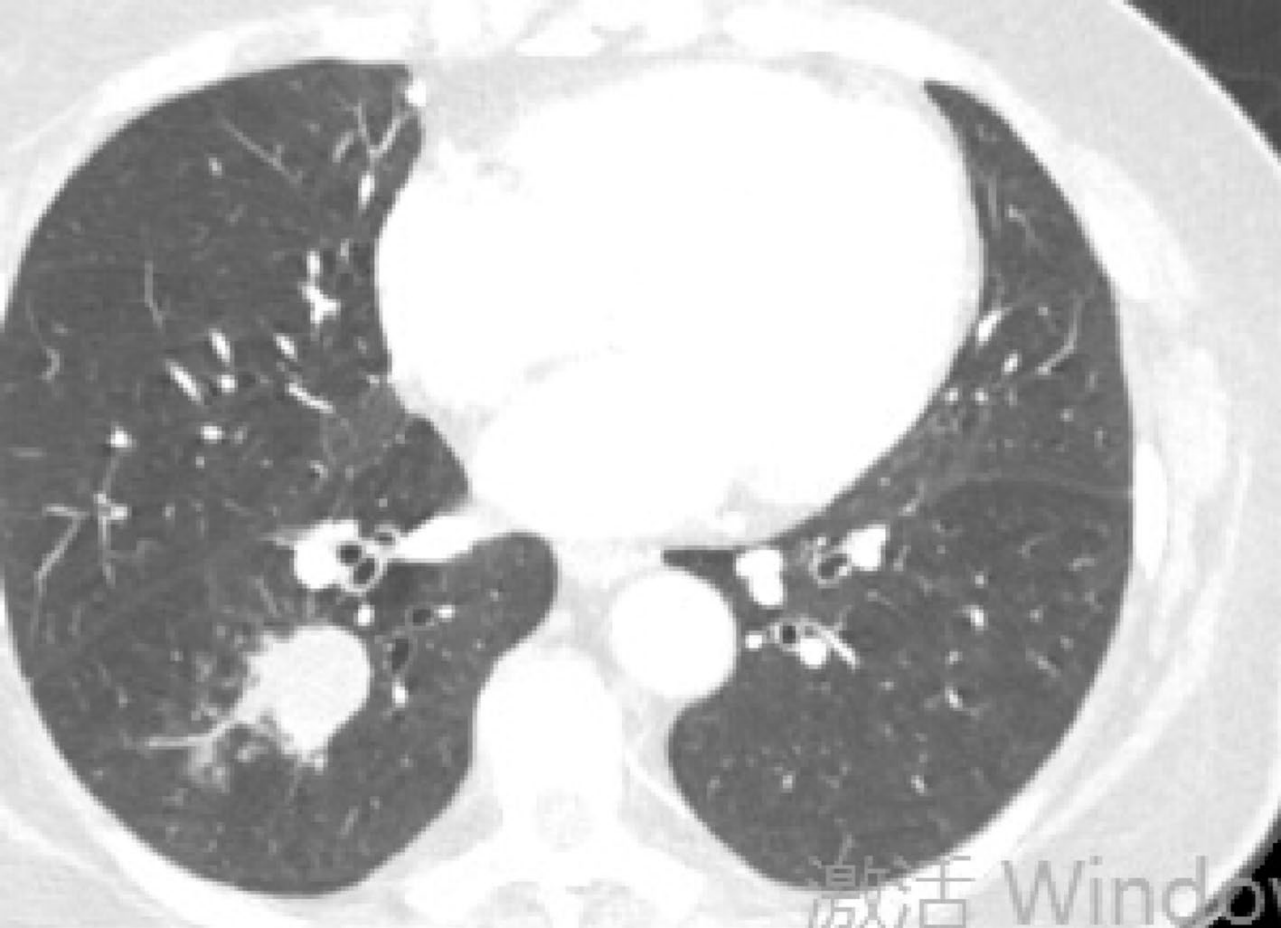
Case1 31 October 2024 CT
